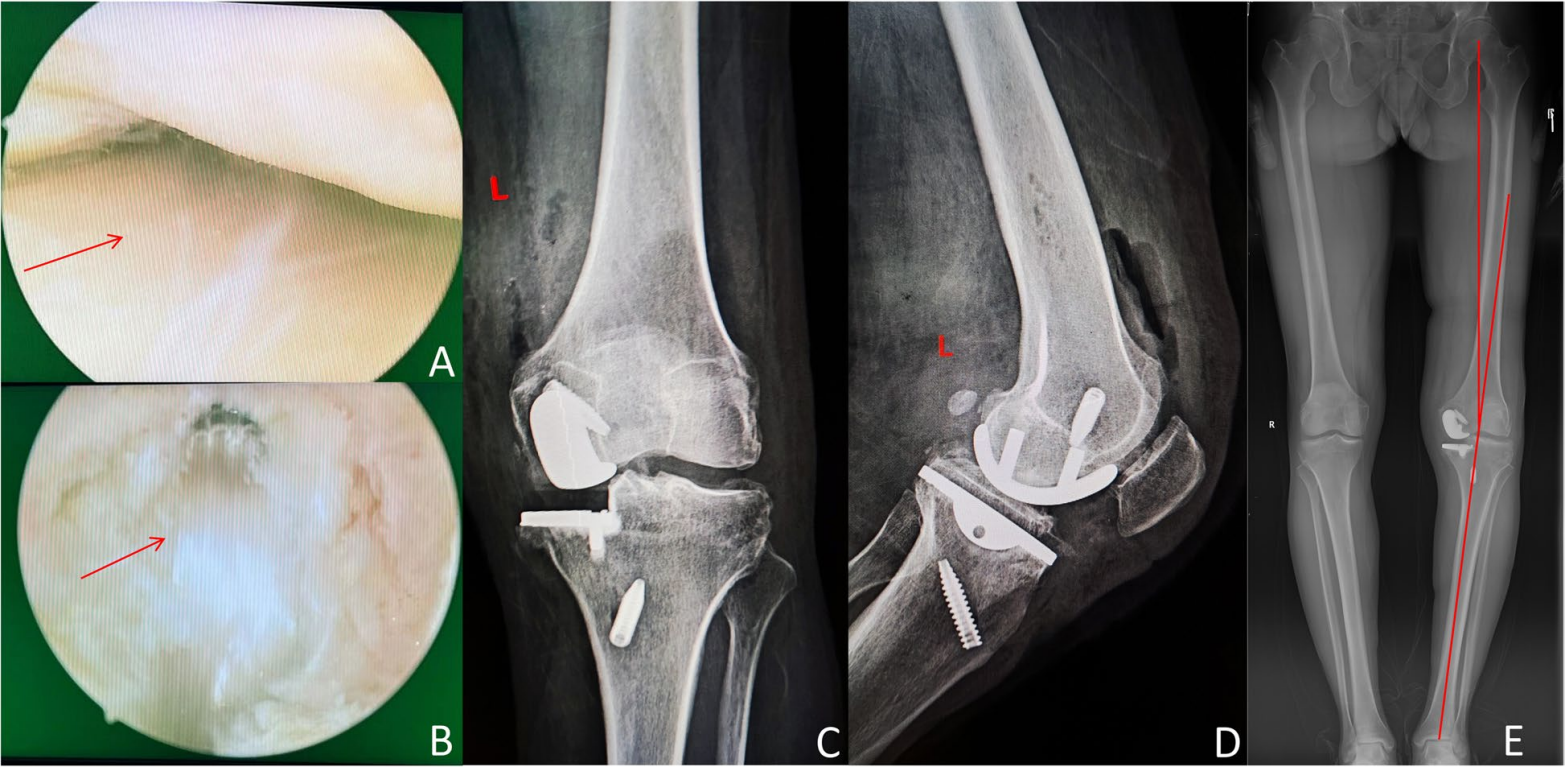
Intraoperative situation and postoperative X-ray. Under arthroscopy, the lateral cartilage was intact (A), and the reconstructed PCL was in the proper position (B). Postoperative X-ray showed that the position of the implant was ideal, and the varus deformity was substantially corrected (C, D and E)
